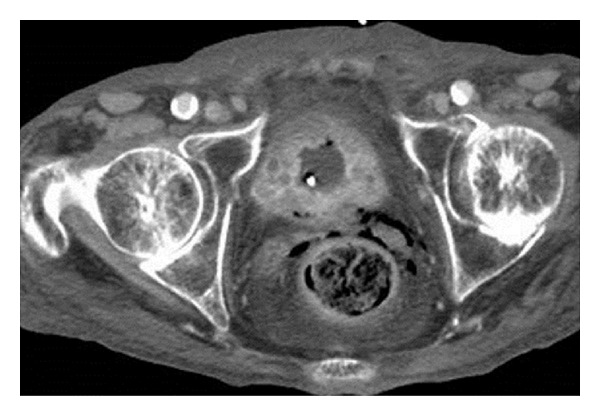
Computed tomography image showing thickening of the urinary bladder wall.
